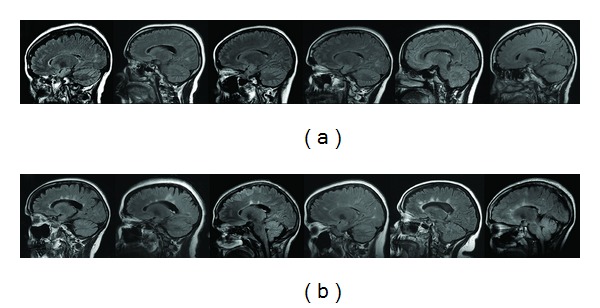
Evaluation of FLAIR sagittal images. Patients with NMO are shown in (a): Dawson's fingers are absent. In contrast, most MS patients (b) demonstrate elongated, flame-shaped lesions oriented along the course of deep medullary veins, compatible with perivenous inflammation.
